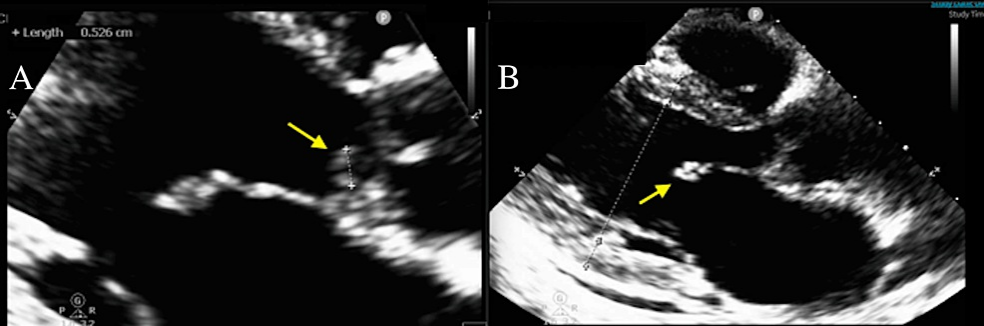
Transthoracic echocardiogram (TTE) long-axis view (A) Arrow indicating aortic valve vegetation. (B) Arrow indicating mitral valve vegetation.
